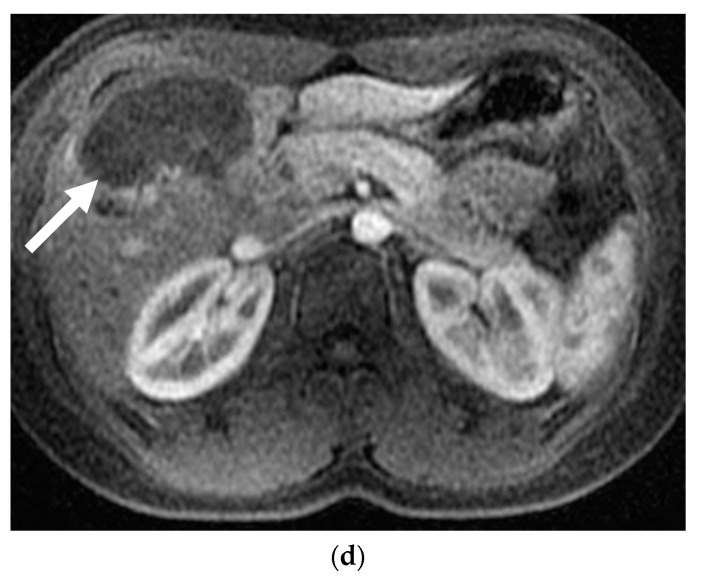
A 24-year-old female with focal nodular hyperplasia (FNH) and right upper quadrant pain. (a) Magnetic resonance imaging (MRI) with contrast demonstrates a hypervascular lesion in segments 4 and 5 (solid white arrow). Twenty-minute delayed images showed continued enhancement (not shown). (b) Angiogram reveals segment 4 and segment 5 arteries that feed a hypervascular mass. Each artery was infused with 200 and 300 µm PVA particles until stasis. (c) Post-embolization angiogram shows no flow to the FNH. (d) MRI with contrast one month after embolization reveals no residual enhancement and a decrease in the size of the FNH (solid white arrow). The patient’s pain resolved following the embolization.
